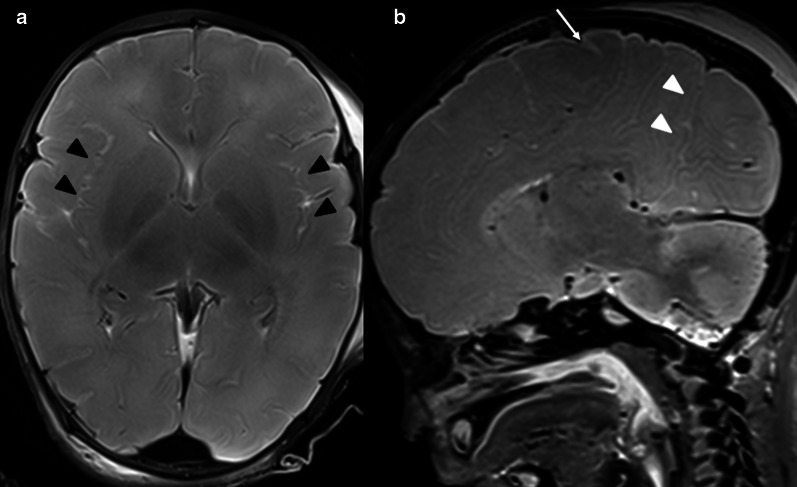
36-week foetus with normal cerebral sulcation. a Axial T2-weighted image shows normal Sylvian fissure (black arrowheads). b Sagittal T2-weighted image shows normal parieto-occipital fissure (white arrowheads) and central sulcus (white arrow)
